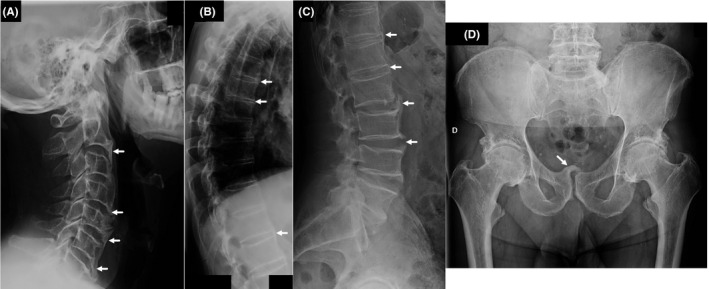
A: Cervical spine radiography revealing thickening and ossification of anterior longitudinal ligament (arrows). B: Thoracic spine radiography revealing ossification of anterior longitudinal ligament (arrows). C: Lumbar spine radiography revealing large nonmarginal osteophytes (arrows). D: Pubic symphysis enthesopathy (arrow)
